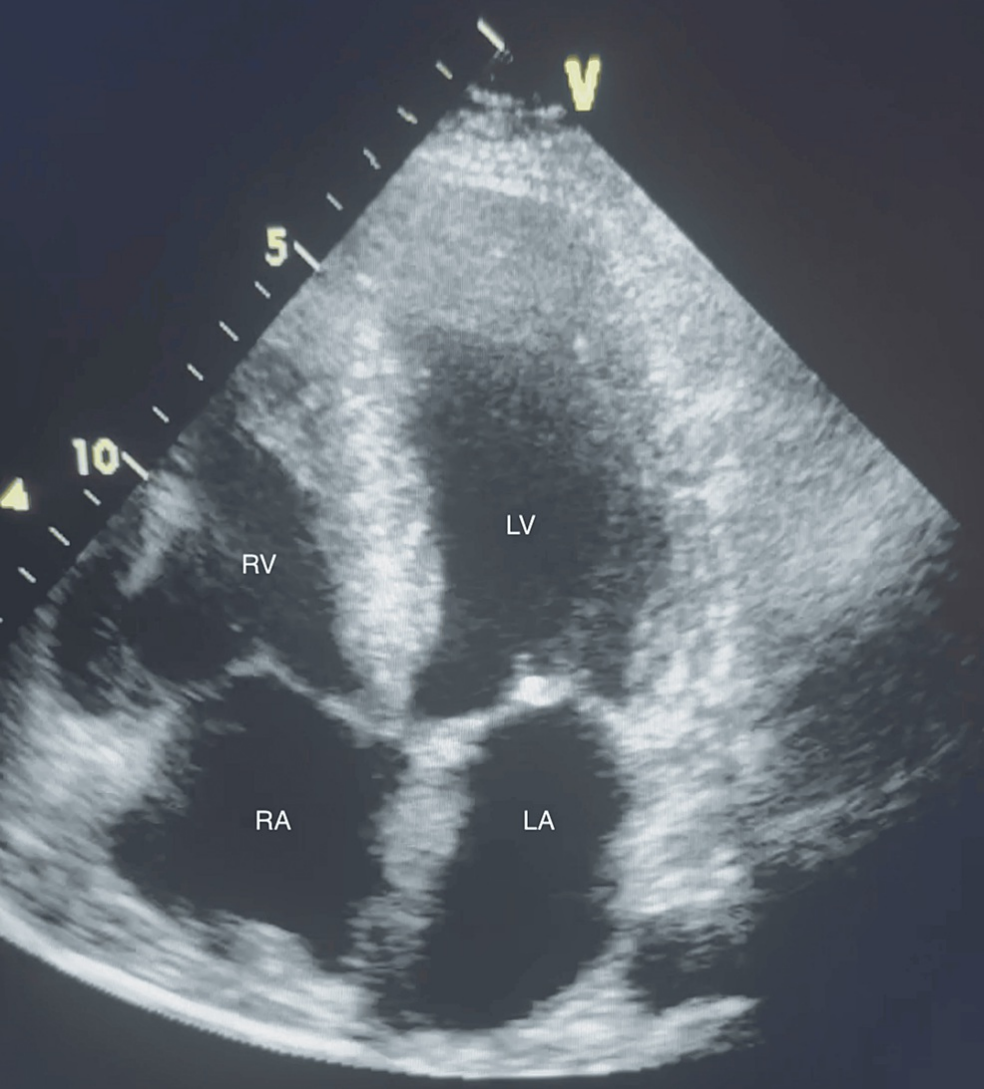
Apical four-chamber view on TTE 11 days prior to presentation, revealing biatrial enlargement LA: left atrium; LV: left ventricle; RA: right atrium; RV: right ventricle; TTE: transthoracic echocardiogram
